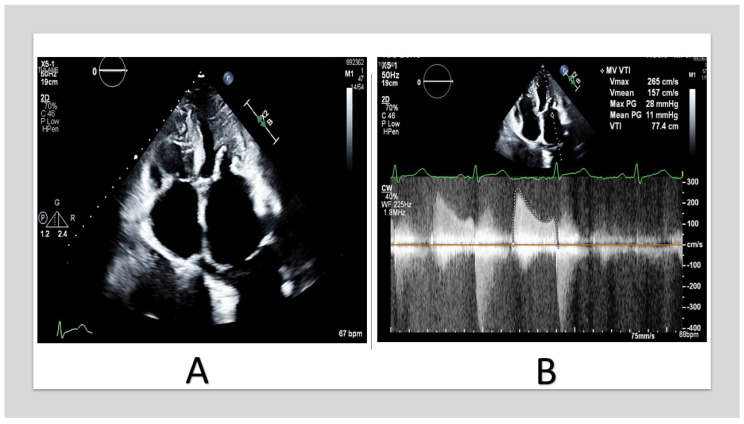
TTE of 57_year_old woman with end-stage renal disease. (A) shows severe MAC involving the anterior and the posterior annulus with extension to the leaflets and the chordae. (B) shows mitral inflow continuous-wave Doppler with mean gradient of 11 mmHg. (TTE: transthoracic echocardiography, MAC: mitral annular calcification).
